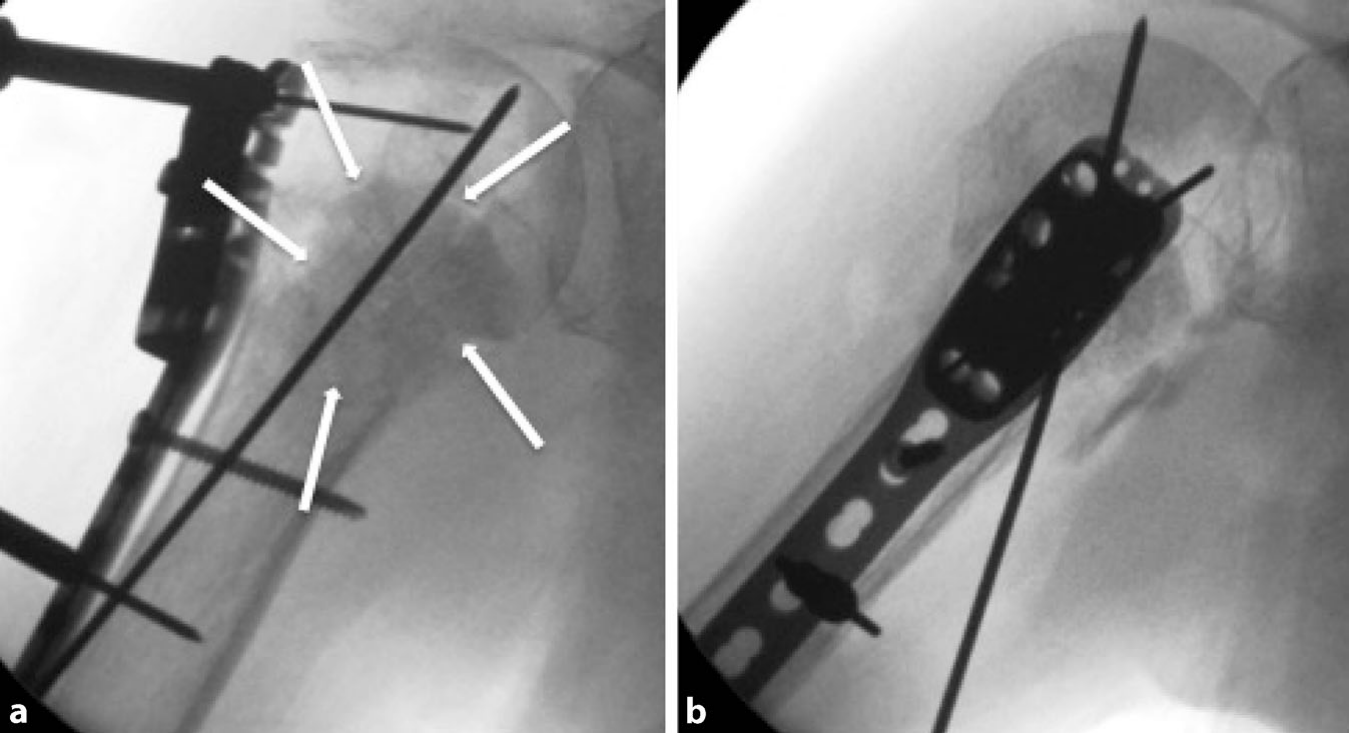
Intraoperative fluoroscopy. Anteroposterior (a) and axial (b) fluoroscopic radiographs. *White arrows* indicate the allograft
